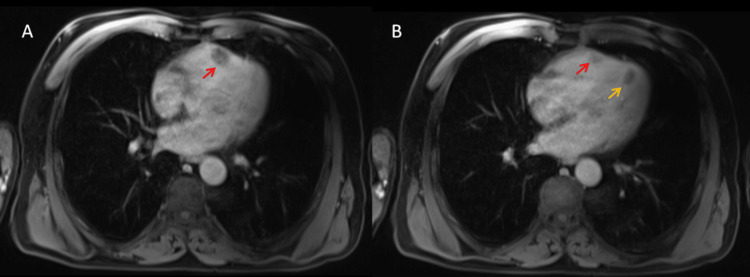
Axial T1-weighted fat-saturated sequence with gadolinium contrast from a cardiac MRI. (A) The right ventricular mass, situated within the anterior myocardial wall, exhibits signal suppression, confirming its fatty composition (red arrow). (B) The left ventricular apical mass shows a similar suppression pattern, further supporting its adipose nature (orange arrow).
